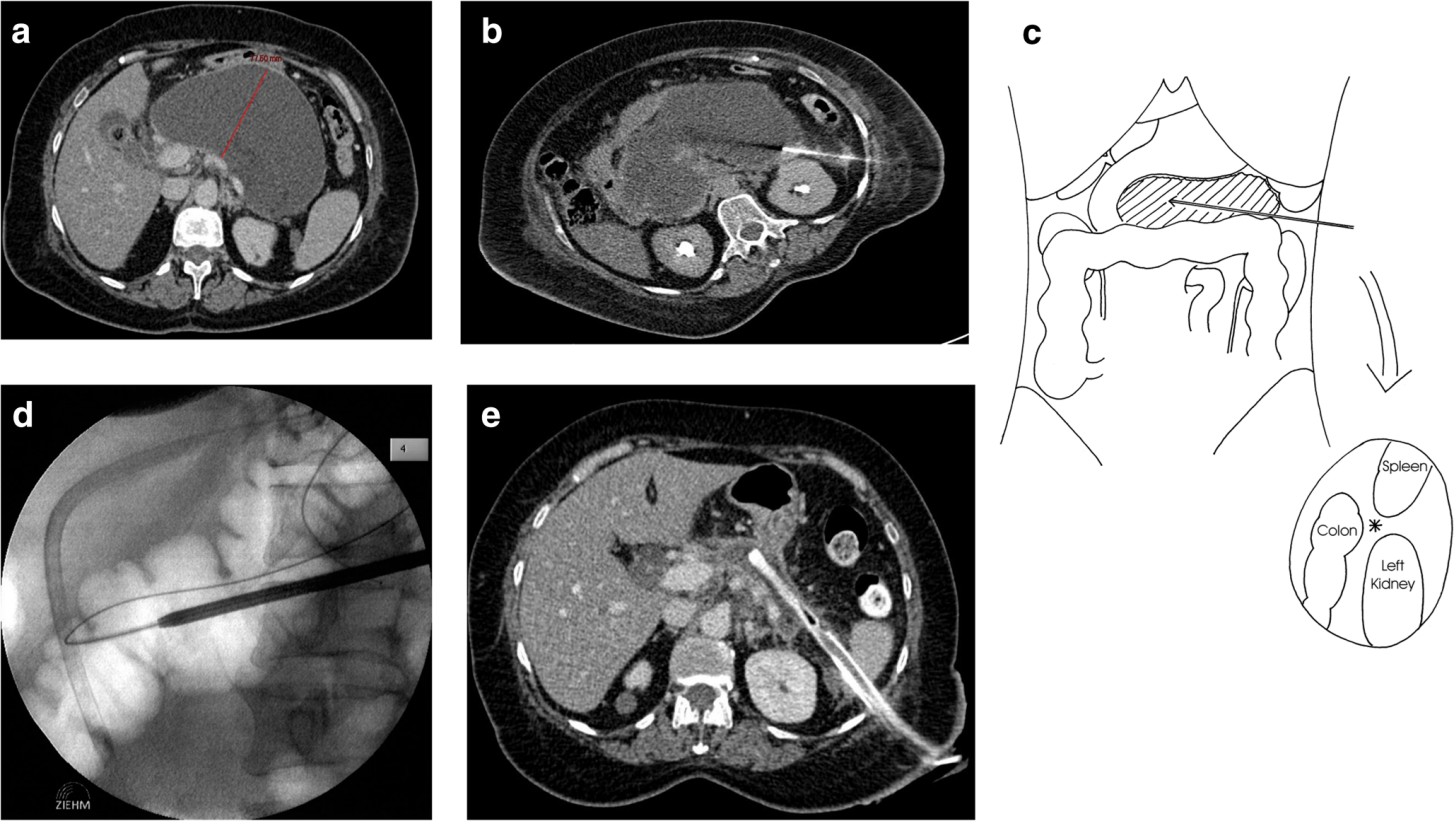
The serial treatment of necrotizing pancreatitis by MARPN (a). In the radiology department, the guidewire is inserted into the center of the necrotic mass, taking a line between the lower pole of the spleen, the splenic flexure of the colon, and the upper pole of the left kidney (b, c). Then in the operating room, the surgeon dilates the guidewire track using increasing diameter nephrectomy dilators, under X-ray control using the vertebral column and the position of a nasogastric tube as a reference point (d). Following multiple skunk procedures using a straight rigid nephroscope, the necrosis has largely been cleared and the necrotic cavity has collapsed around the 28-French chest drain (e); the tract will heal by granulation tissue on steady withdrawal then downsizing of the drain over several weeks as an outpatient
